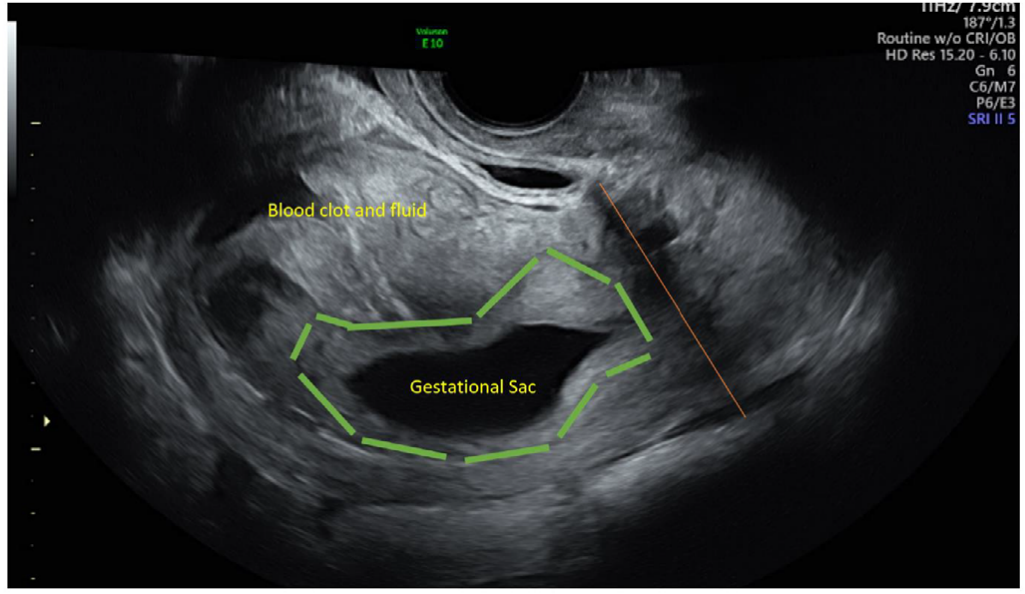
Mid‐sagittal view of the uterus at 8 weeks 3 days of gestation (gestation based on initial dating ultrasound). The upper cavity is distended by heterogeneous material suggestive of intracavitary fluid and blood. The elongated and irregular outline of the gestational sac can be seen in the lower cavity, above the level of the internal os with a surrounding decidual reaction (outlined in green). There is no significant pelvic free fluid. The orange line indicates the level of the internal os.
